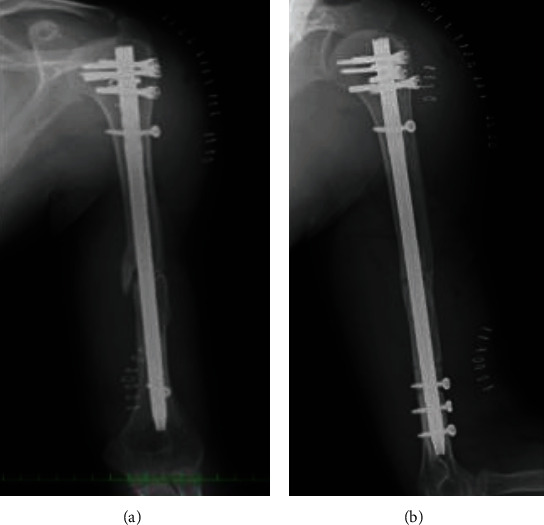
Radiographs just after the nonunion surgery was performed at our hospital. The gap between bone fragments seemed to be reduced by compression ((a) before nonunion surgery and (b) after the nonunion surgery). The compression screw appeared to be curved.
